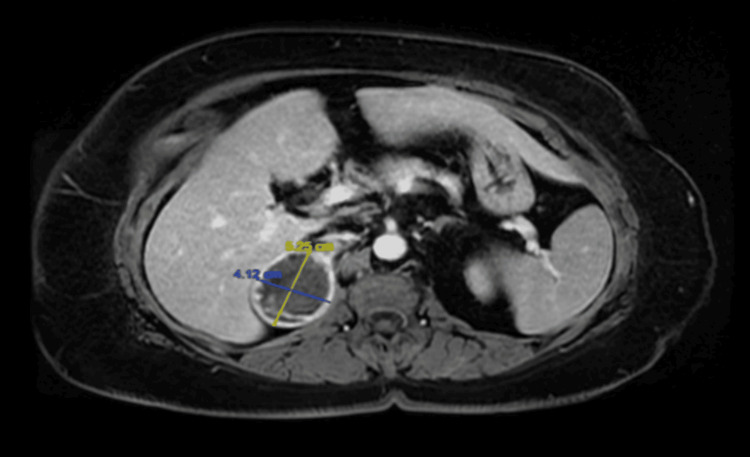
Transverse section of MRI abdomen showing the maximum dimension of the right adrenal mass (T2-weighted)
